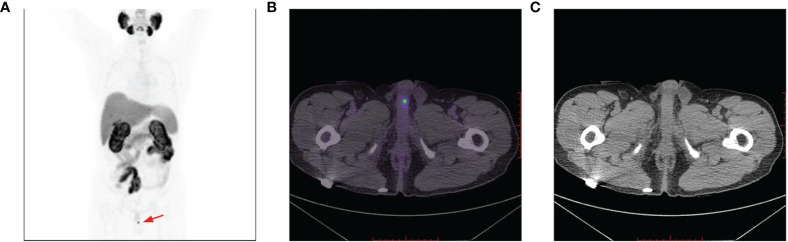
A ^18^F-PSMA-1007 PET/CT avid solitary penile lesion with a SUV_max_ of 6.4 (A, B); no morphological abnormalities of the penis detected on CT imaging (C).
